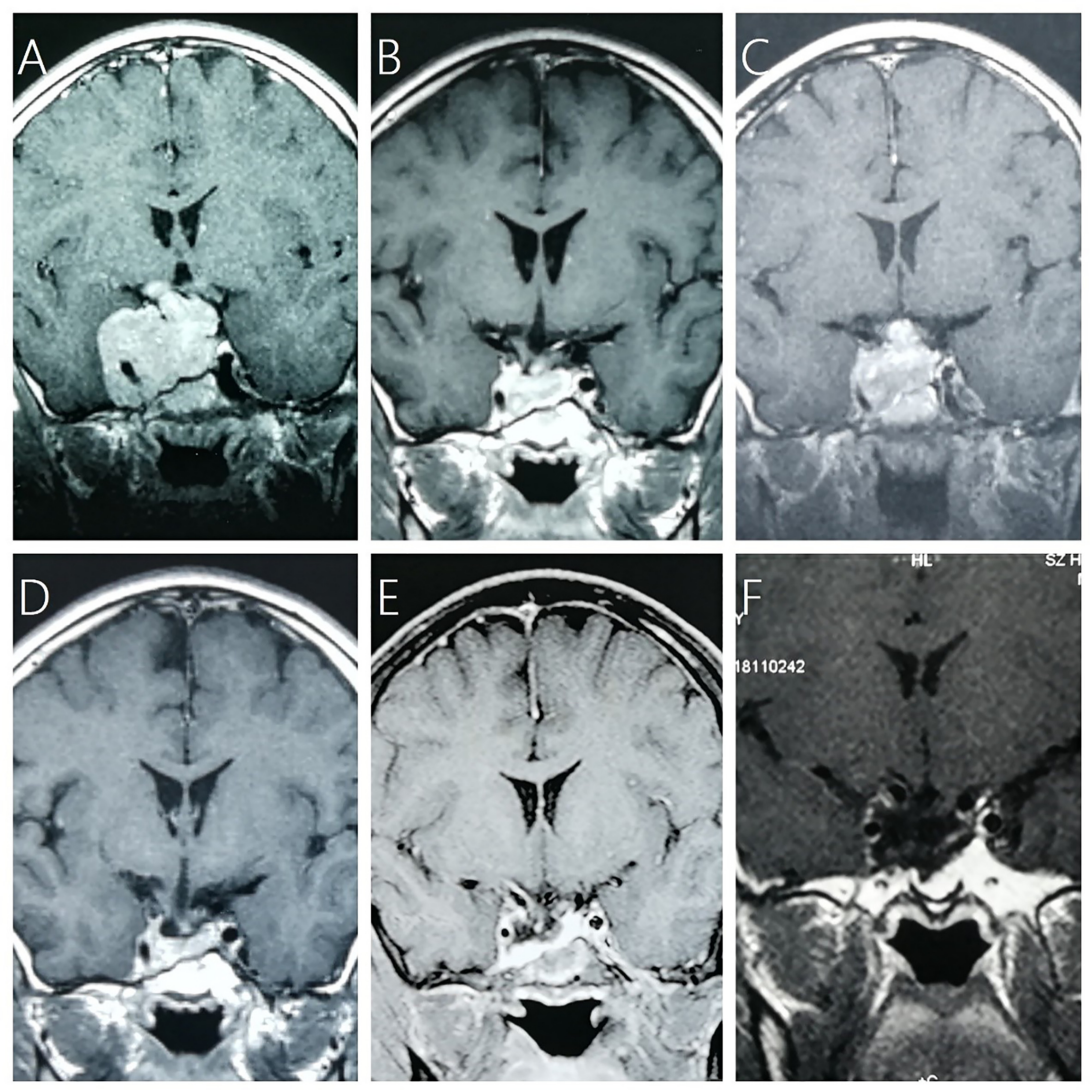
A 13-year-old boy with NFPA (max diameter of 7.6 cm) received adjuvant GKRS (10 Gy/35%) after subtotal resection and repeat GKRS (12 Gy/35%) for tumor regrowth at 36.5 months after prior GKRS. (A) contrast-enhanced coronal T1-weighted magnetic resonance imaging (MRI) scans showed residual giant NFPA after surgical resection. (B) MRI showed tumor shrinkage at 24.6 months after GKRS. (C) MRI showed tumor regrowth at 37.9 months after prior GKRS. (D) MRI showed tumor shrinkage at 10.1 months after repeat GKRS. (E) MRI showed tumor shrinkage at 68.8 months after repeat GKRS. (F) MRI showed tumor shrinkage at 205.0 months after repeat GKRS.
